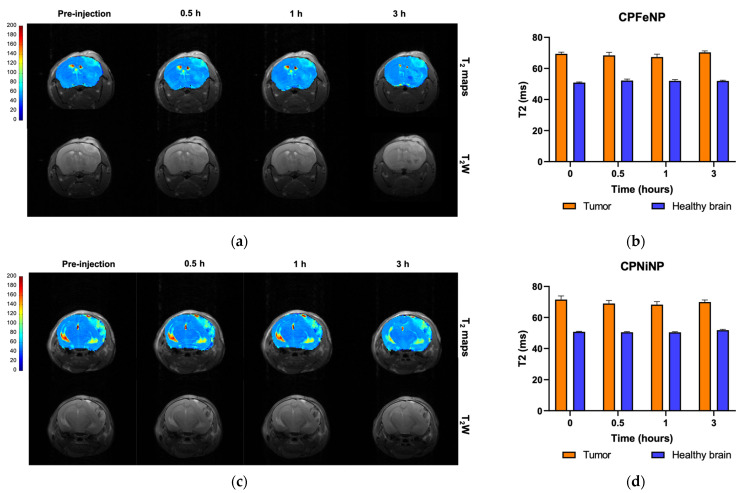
T_2_ color-code based maps and T_2_W images of orthotopic GBM model. Data were acquired before the i.v. administration of the IONP-doped CPNs, and at 0.5, 1 and 3 h after the injection. MRI evaluation of CPFeNP (a) and CPNiNP (c). Graphical bar showing the variation in T_2_ values (mean ± SEM) along the temporal evaluation of CPFeNP (b) and CPNiNP (d).
